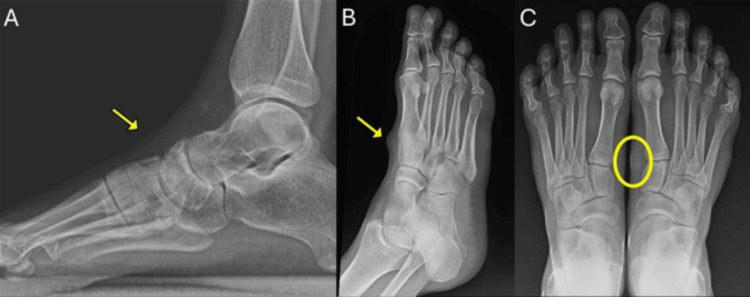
Weight-bearing lateral (A) and dorsoplantar oblique (B) radiographs of the right foot, and dorsoplantar view of both feet (C). The yellow arrows indicate the area of small soft-tissue mass with increased soft-tissue density adjacent to the right first tarsometatarsal joint. Image C highlights asymmetry between the right and left midfoot regions, with soft-tissue prominence on the right (circled). No evidence of bone involvement, periosteal reaction, or adjacent osseous destruction. No intra-articular abnormalities or calcifications were identified. The lateral radiograph was cropped to enhance visualization of the soft-tissue mass.
